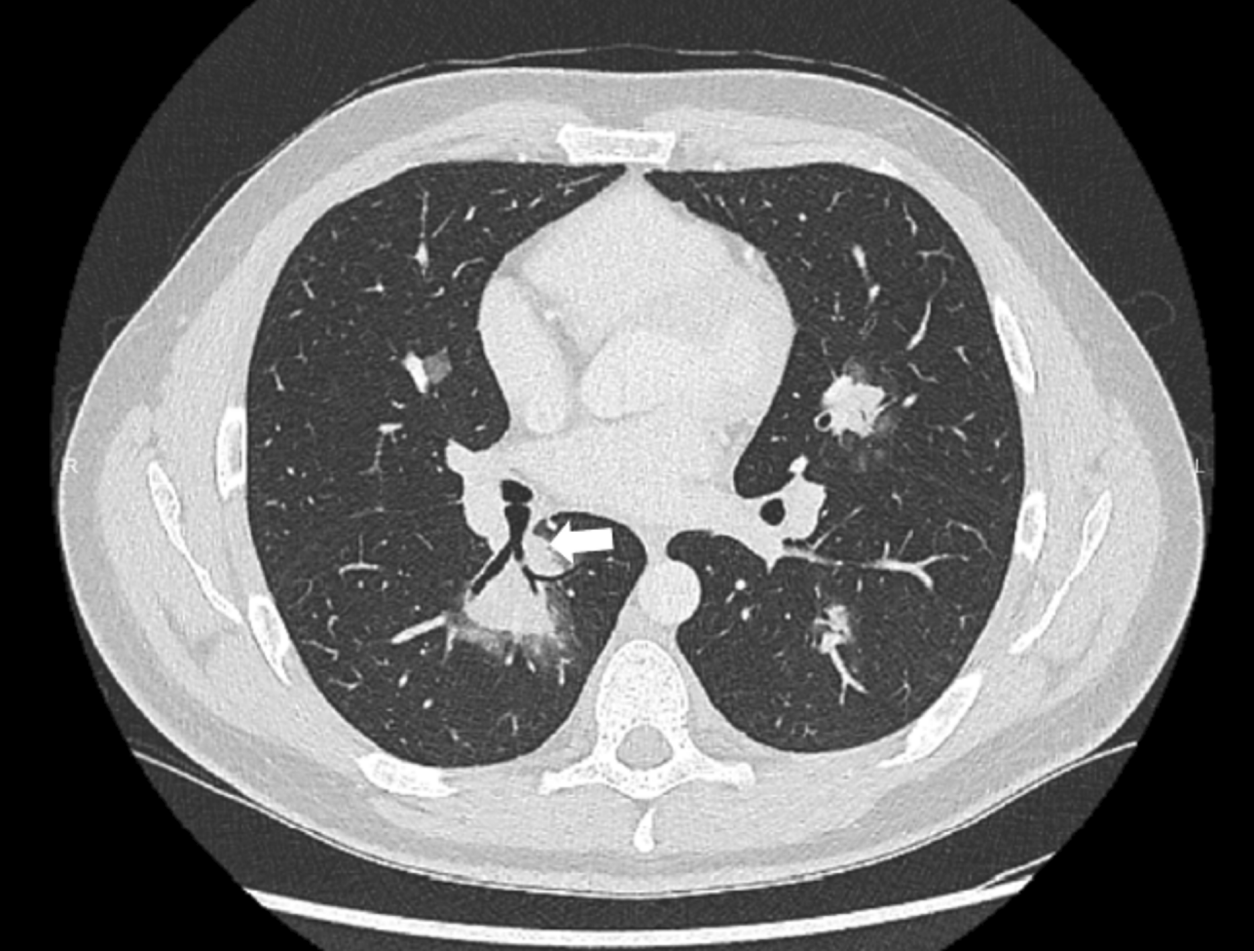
Chest CT scan showing multiple consolidation with peripheral halo sign. The white arrow indicates the target of EBUS‐TBNA and EBUS guided cryobiopsy.
